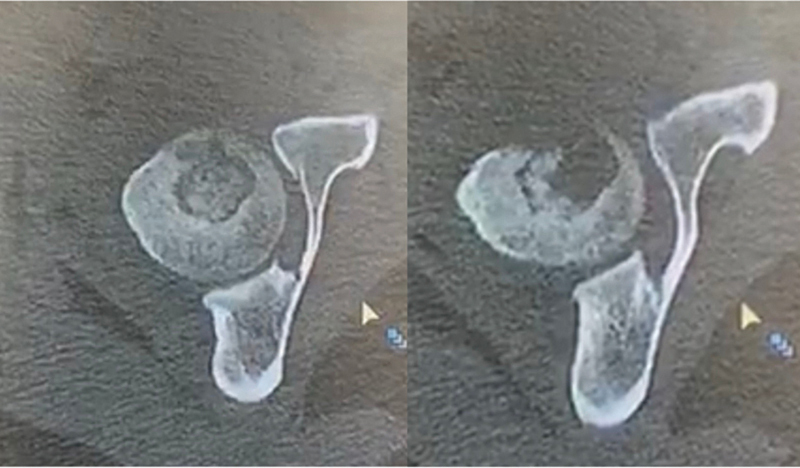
Initial axial images of the CT-scan demonstrating cortical erosion and a cavitary lesion.
